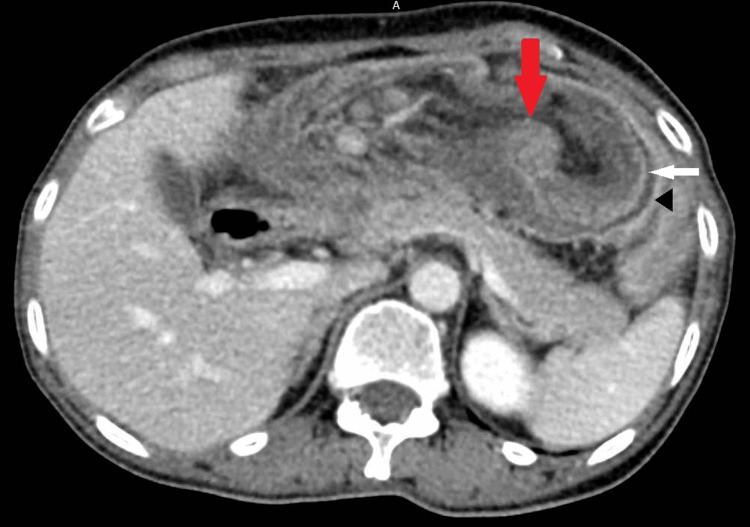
Representative image from preoperative CT scan showing the cecum intussuscepted into the colon. The red arrow points to the cecal tumor. The white arrow points to the cecal bowel wall, intussuscepted up to the splenic flexure (noted by the black arrowhead). CT: computed tomography
